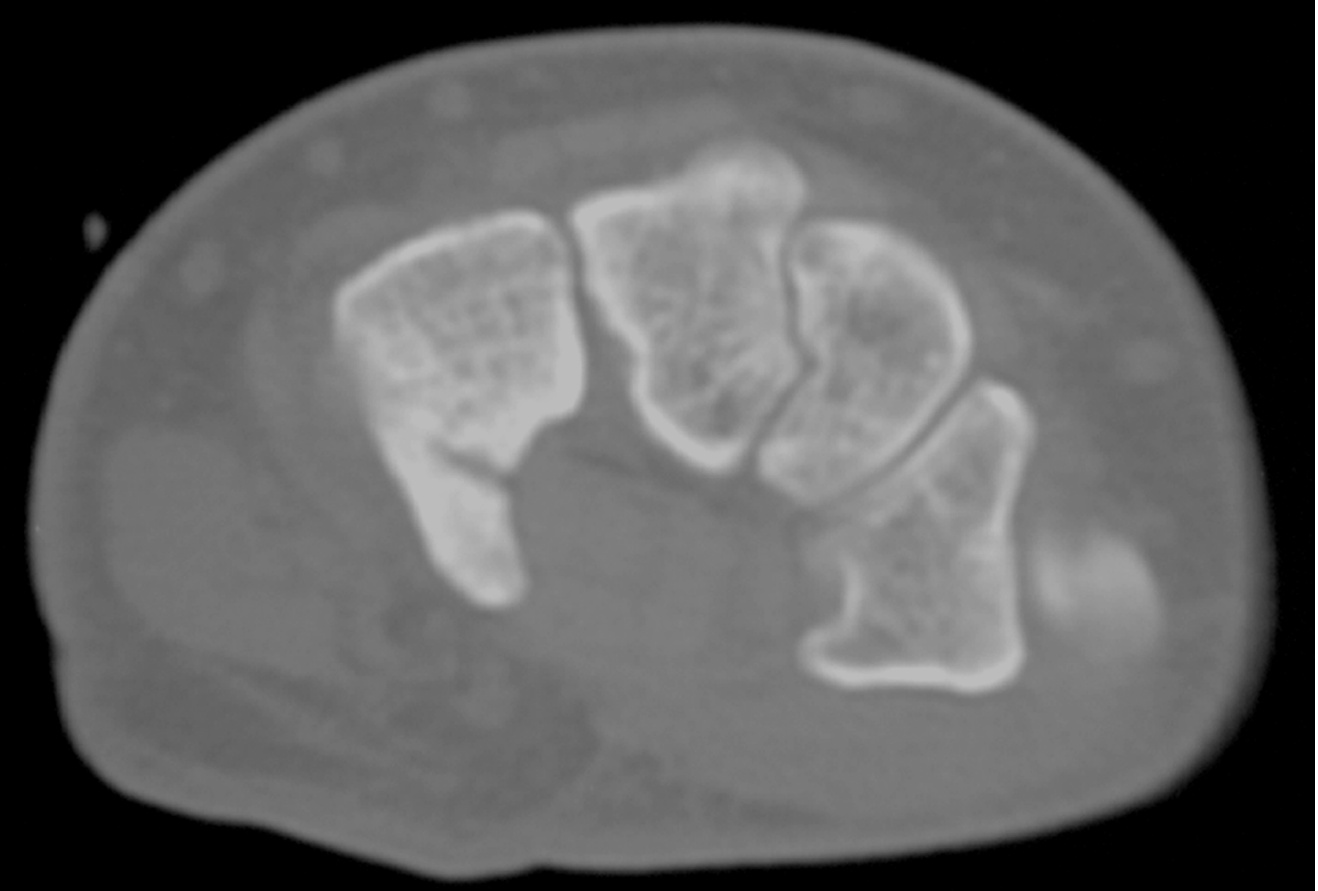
CT of left wrist indicating hamate hook fracture.
